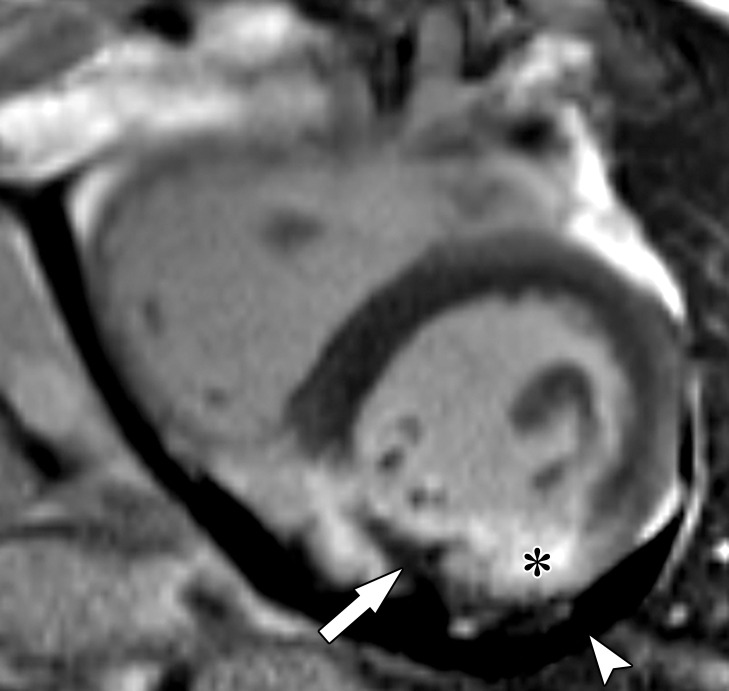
Intramyocardial hematoma in a 60-year-old man with a history of MI. Recent echocardiographic findings (not shown) were suggestive of expanding posterior wall intramyocardial hemorrhage and dissecting intramyocardial hematoma after an acute MI.(a) Short-axis phase-sensitive inversion-recovery LGE image shows a transmural MI (*), with an area of nonenhancing intramyocardial hematoma (arrow). Pericardial effusion is also depicted (arrowhead).(b) Coronal CT image shows contrast material infiltration into the myocardial wall (arrow). Moderate pericardial effusion (*) is present.*LA* = left atrium,*RA* = right atrium.
